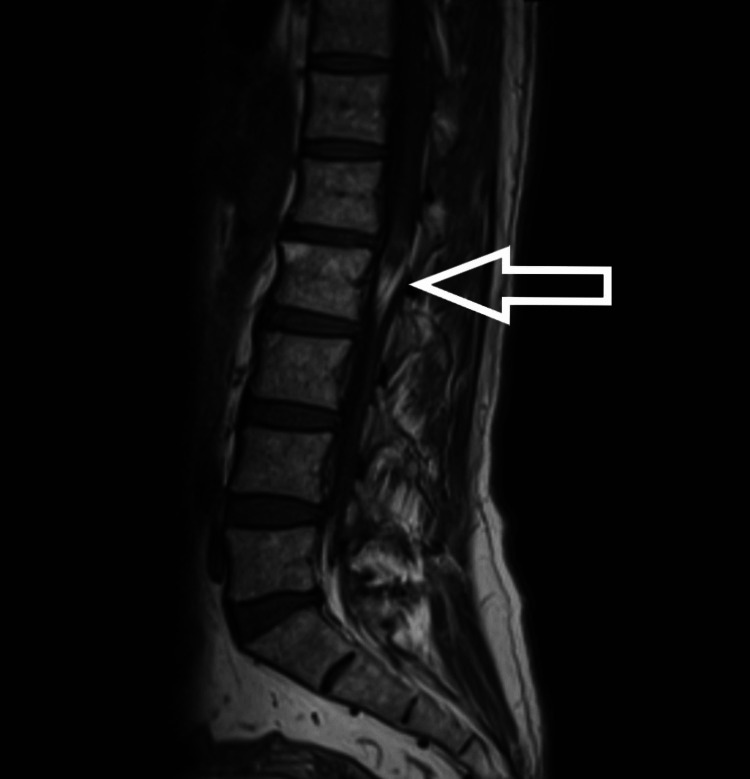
T1-weighted MRI (sagittal) showing hyperintense intramedullary mass
